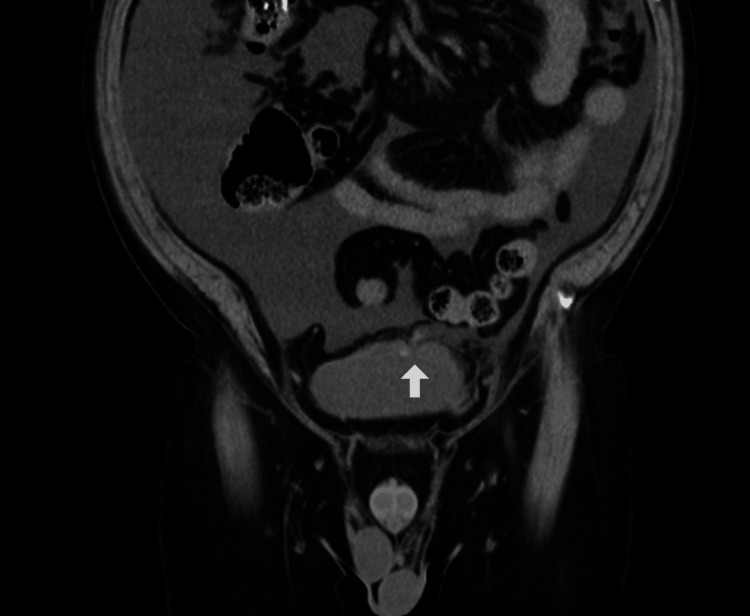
Abdominal coronal CT scan on admission. The image reveals a communication between the bladder apex and the peritoneal cavity (arrow).
